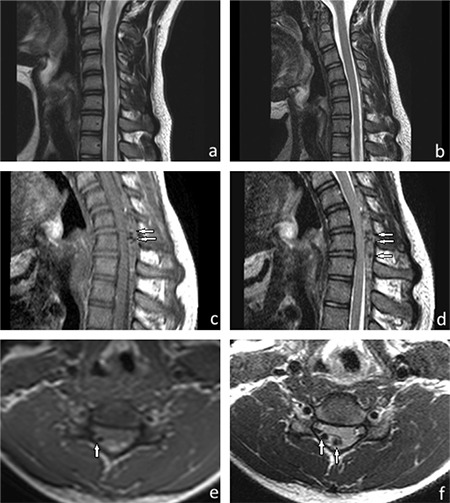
a-f. Hirayama disease. Normal magnetic resonance imaging findings on the sagittal T2-weighted images in a neutral position (a) and in slight flexion (b). However, dolichoectatic vascular structures (arrows) are seen on the sagittal T1-weighted image (c), sagittal T2-weighted image (d), axial T1-weighted image (e), and axial T2-weighted image in increased flexion (f). Prominent posterior epidural space and spinal cord compression are present between the C5 and D1 levels (c-f).
